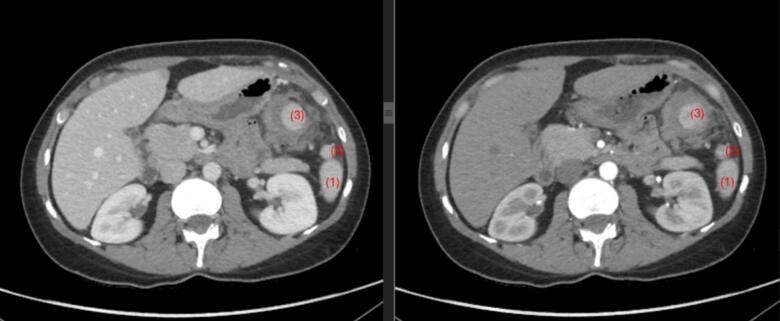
Axial cuts of the follow up abdominal CT scan with contrast injection: portal phase on the left side and arterial phase on the right side. (1) Represent the caudal main spleen, (2) represent the unremarkable accessory spleen, (3) represent the ruptured accessory spleen with interval further decrease in the surrounding evolving hematoma.
